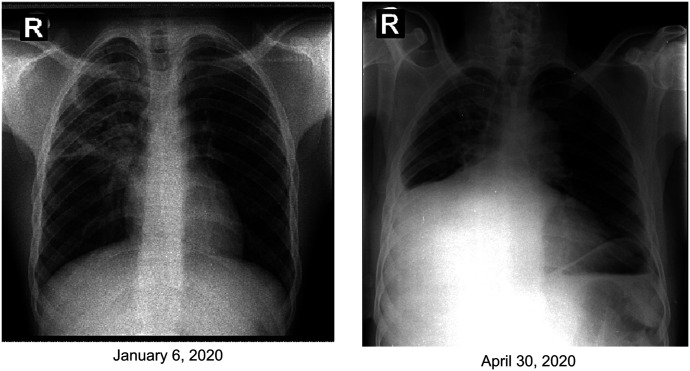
Chest radiograph at first presentation and when coinfected with SARS-CoV-2.
